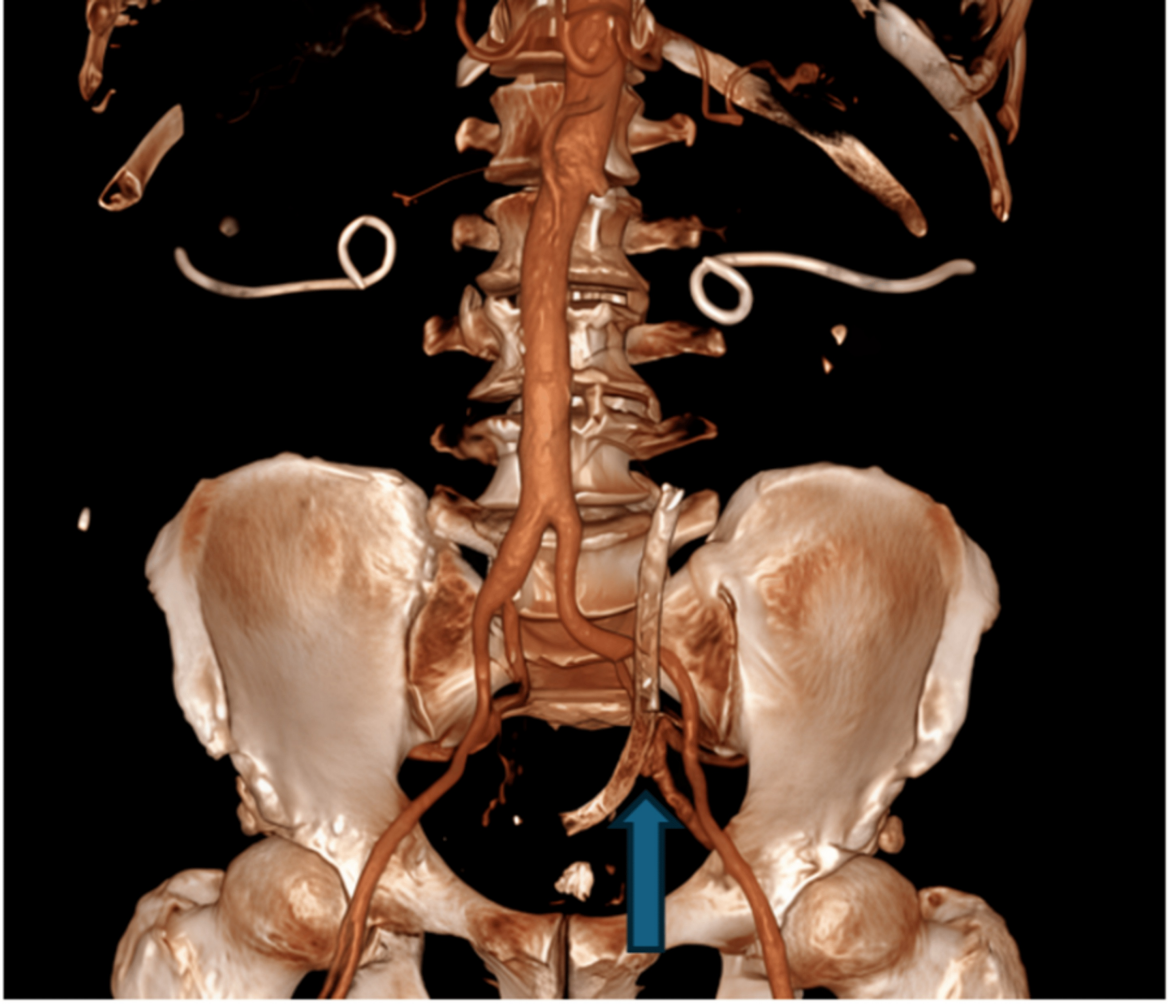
3D CT angiography showing left internal iliac artery aneurysm adjacent to ureteric metallic stent (blue arrow)
